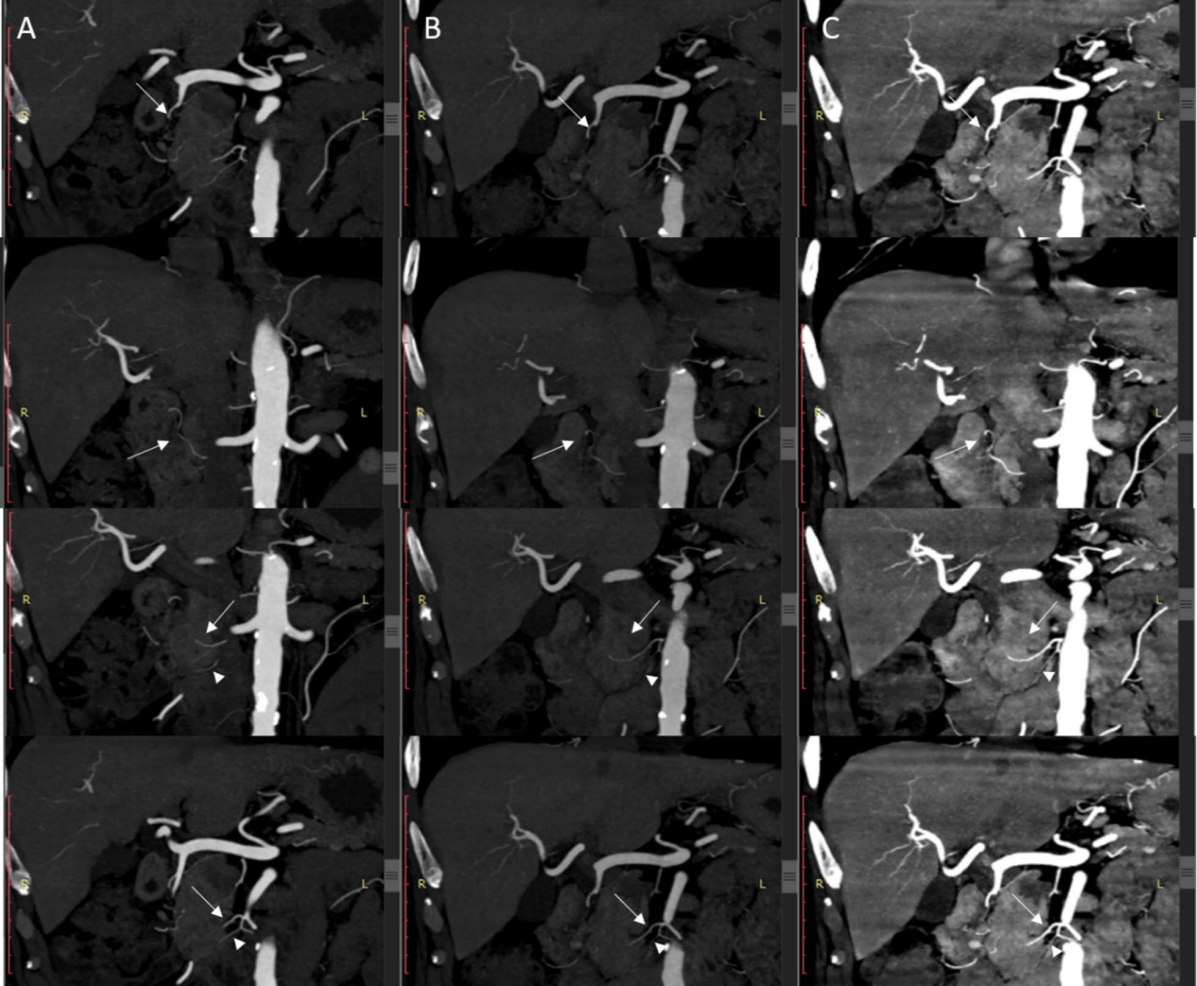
Depiction of pancreatic arteries in ND-CM, LD-CM, and VMI+ LD-CM images. a–c The posterior pancreaticoduodenal artery arch of one patient who underwent ND-CM (0.8 mL/kg, 400 mgI/mL, and 320 mgI/kg), LD-CM (0.56 mL/kg, 400 mgI/mL, and 224 mgI/kg), and VMI+ LD-CM using the same window level (300 HU) and width (600 HU). The CT value of the aorta was highest on VMI+ LD-CM images (c), lower on ND-CM images (a), and lowest on LD-CM images (b). The posterior pancreaticoduodenal artery arch (white arrow) can be depicted clearly on all images. The anterior inferior pancreaticoduodenal artery (AIPDA) (arrowhead) on LD-CM images (b) cannot be seen clearly, which showed a faint display on VMI+ LD-CM images (c) and can be seen clearly on ND-CM images (a). *ND-CM* normal-dose contrast medium, *LD-CM* low-dose contrast medium, *VMI*+ advanced virtual monoenergetic imaging
